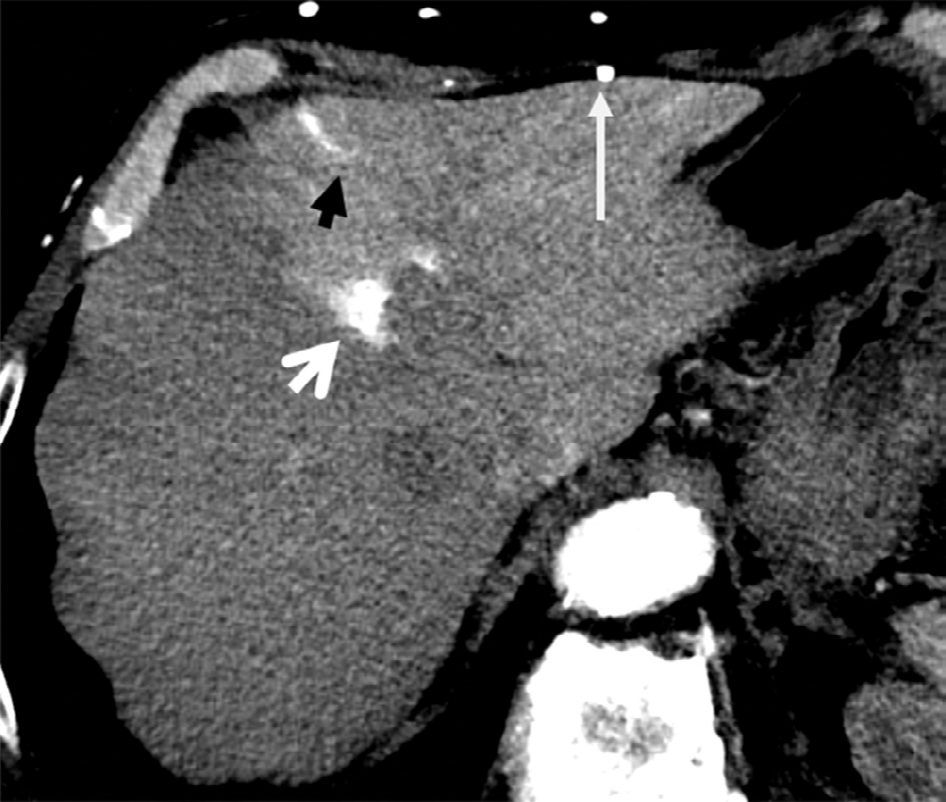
Axial liver CT showing enhancing area in liver segment 4A.
